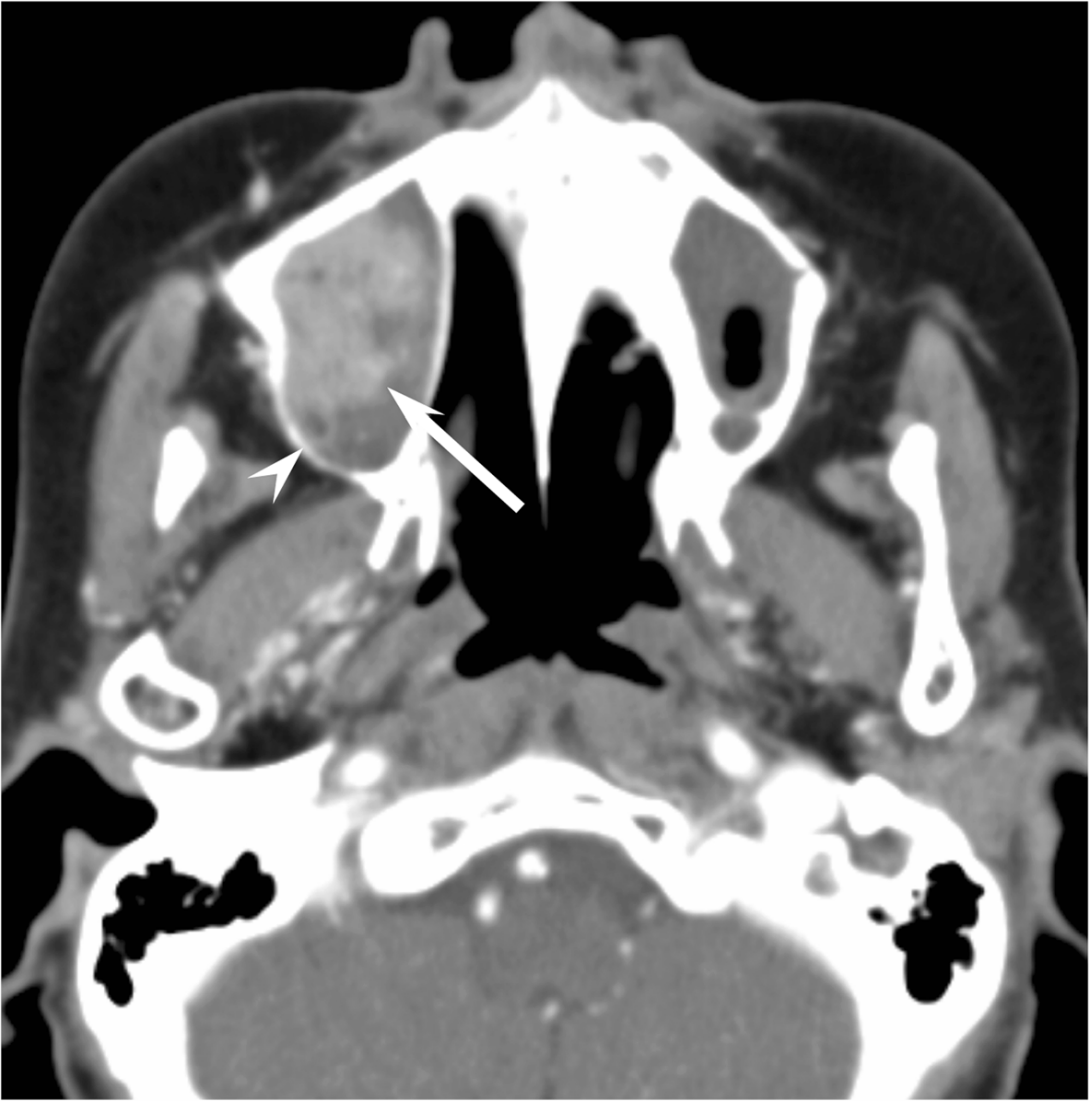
A 73–year-old woman with maxillary sinus squamous cell carcinoma (expansile-type). Enhanced CT image shows a heterogeneously enhanced mass of right maxillary sinus (arrow). The expansion and erosion of right posterior sinus walls is observed without bony defect (arrowhead)
